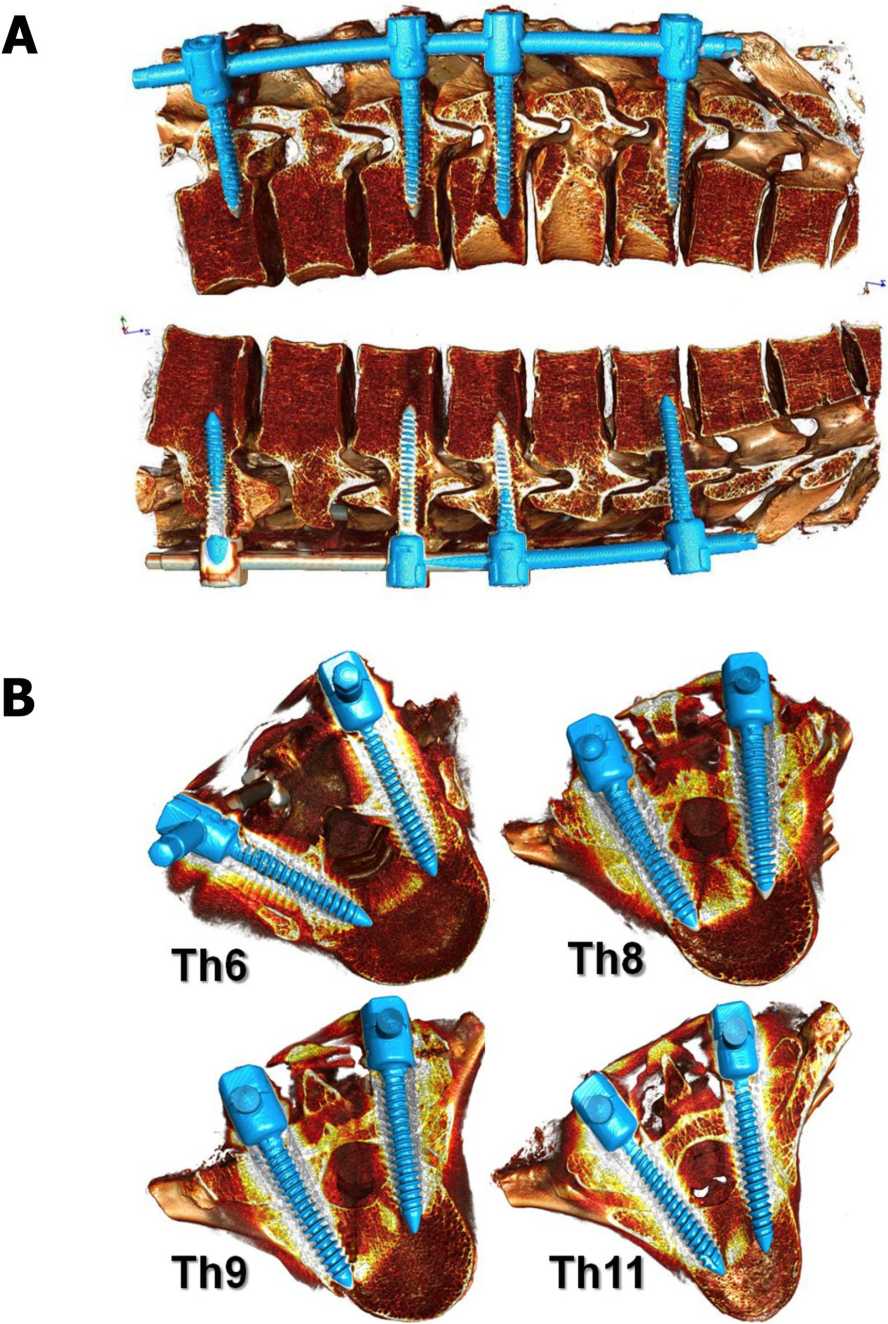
CT evaluation of tested section of the thoracic spine. A) Sagittal CT planes after the experiment—pedicle screws position on the left (upper) and right (lower) figure. B) Axial CT plane of Th6, 8, 9, 11 vertebrae after the experiment.
